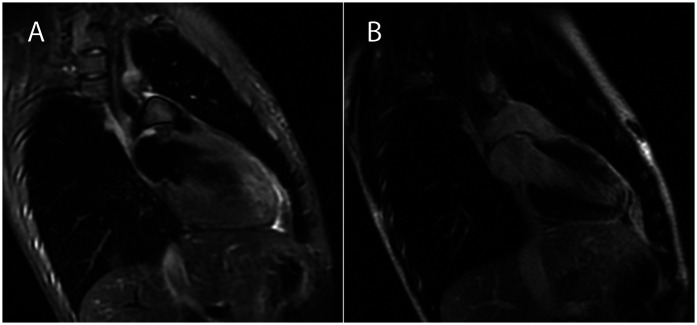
Cardiac magnetic resonance imaging performed 1 month after acute myocarditis onset, two-chamber view: T2 weighted imaging (A) enables visualization of myocardial edema in the anterior wall concordant with regional late gadolinium enhancement (B) with an epi and mid-myocardial distribution.
